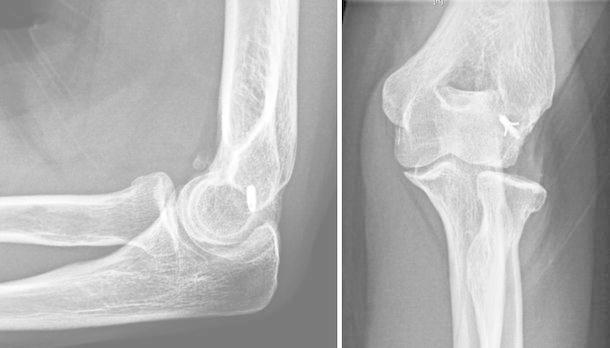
Radiographic examination after lateral epicondylectomy and reattachment of the lateral ligament complex with transosseous fixation and suture anchors
